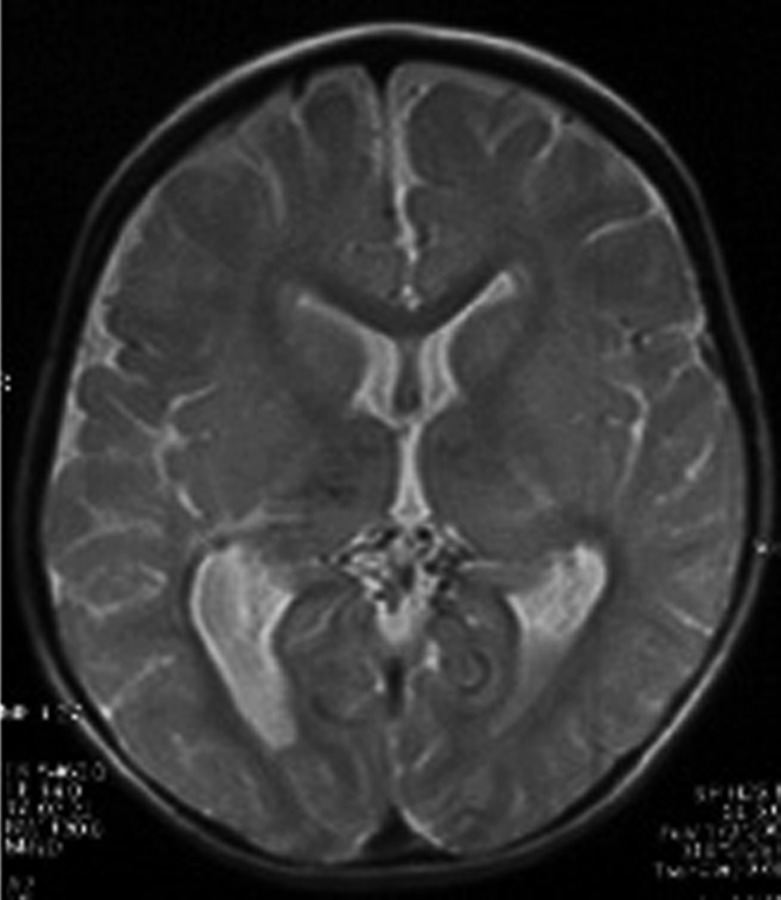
Axial proton density spin echo MRI shows abnormal tiny tubular structures with signal void suspected as small collaterals in the supracerebellar and retropulvinar cisterns associated with similar findings in both thalami
